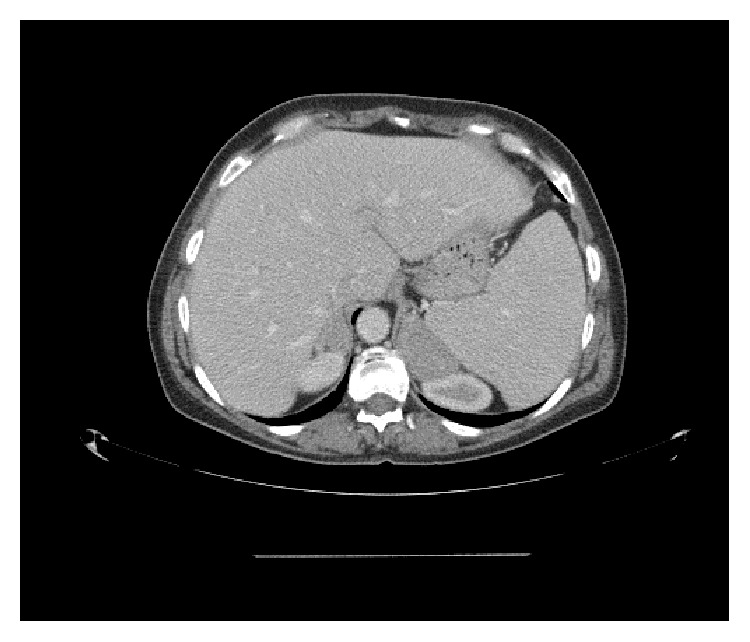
CT of the abdomen showing bilateral, homogenous adrenal gland enlargement.
